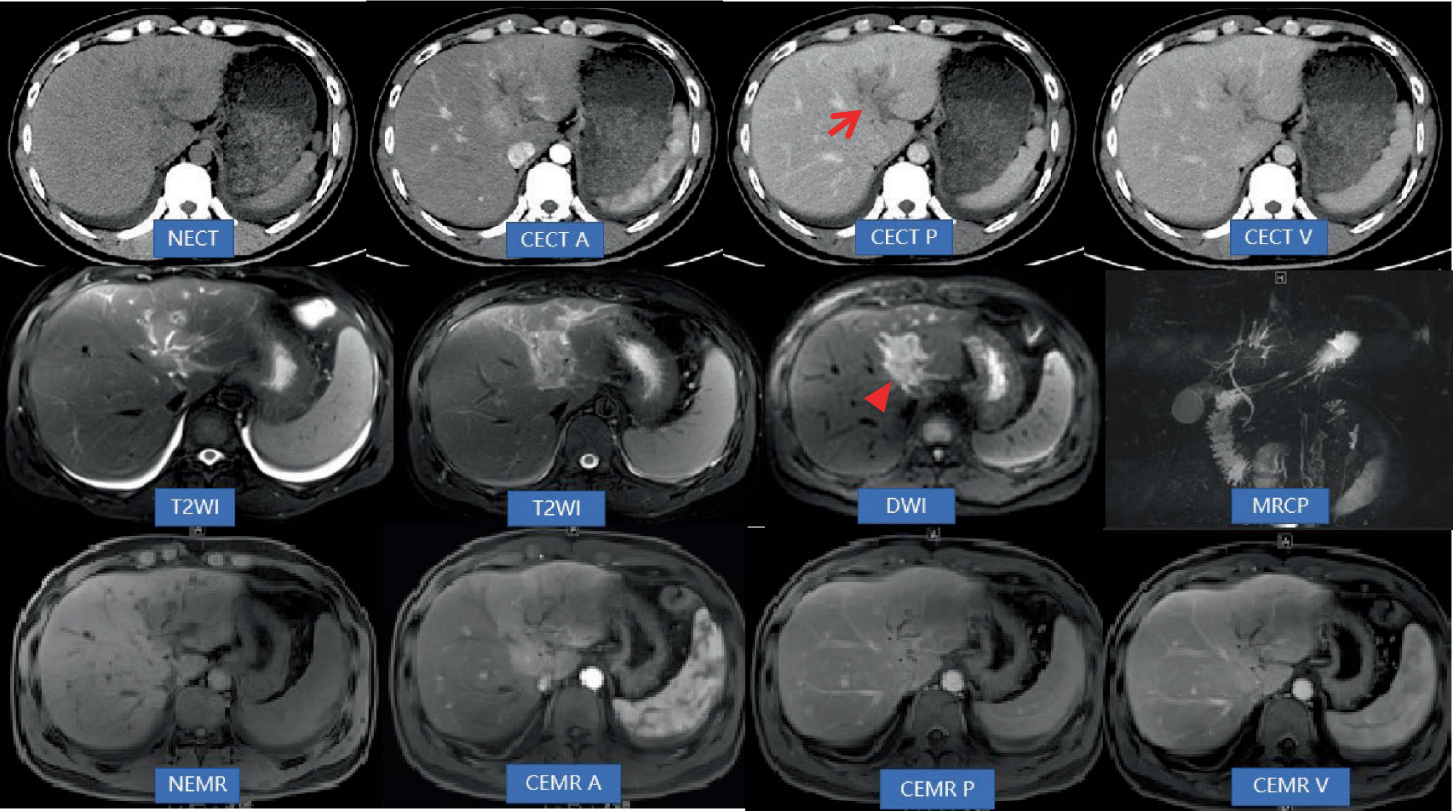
Abdominal non-contrast-enhanced computed tomography (NECT) and contrast-enhanced computed tomography (CECT; A: Artery phase, P: Portal vein phase, V: Vein phase) revealed mild dilatation of the bile ducts in the left lobe of the liver. Additionally, low-density areas (indicated by an arrow) were observed along the hepatic longitudinal fissure, extending toward the hepatic hilum. The boundary of these inflammatory areas appeared unclear, accompanied by narrowing and indistinctness of the adjacent left branch of the portal vein. Inflammatory exudation was also present in front of the colon. Moreover, there was thickening of the peritoneum and omentum. During CECT scans, the lesions exhibited gradual and significant enhancement, displaying a consistent and uniform enhancement pattern. However, the density of the lesions at each stage was lower compared to that of the liver parenchyma. Abdominal non-contrast-enhanced MRI (NEMR) and contrast-enhanced MRI (CEMR; A: Artery phase, P: Portal vein phase, V: Vein phase) demonstrated that the inflammatory areas in Segment 3, near the hepatic hilum, appeared as areas with low signals on T1-weighted imaging and high signals on T2-weighted imaging. On diffusion-weighted imaging (b = 800), limited diffusion was observed within the lesions (indicated by a triangle). These lesions measured approximately 3.6 cm × 3.8 cm and displayed a crab-like edge, distinctly separate from the hilar bile duct and blood vessels. Regarding contrast enhancement, the lesions exhibited moderate progressive enhancement on CEMR. Furthermore, lymph nodes in the hilar region were found to have distinct and uniform enhancement on CEMR.
